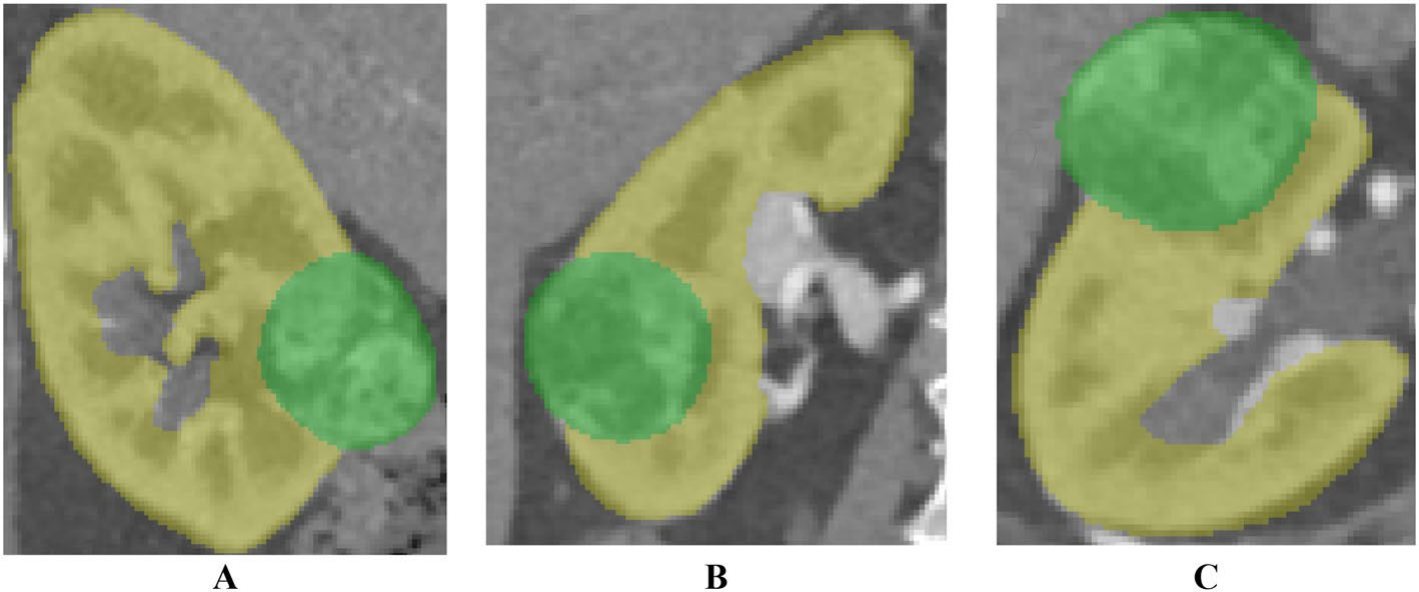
Segmented parenchyma and tumor (A) sagittal, (B) coronal, and (C) axial views from multi-detector computed tomography images of a 61-year-old female patient diagnosed with renal cell carcinoma (RCC).
